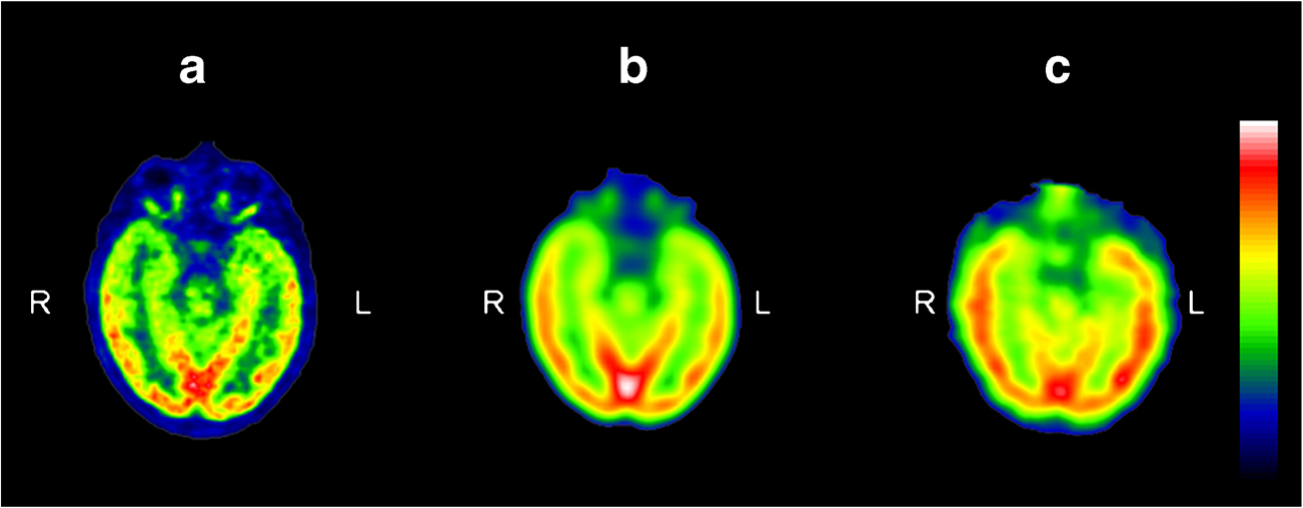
Reprocessed FDG PET images. Axial images: a original FDG PET, b reprocessed FDG PET (rFDG PET), c interictal SPECT. All images are coregistered with MRI images. The *colour scale* indicates intensity values. *L* left, *R* right
